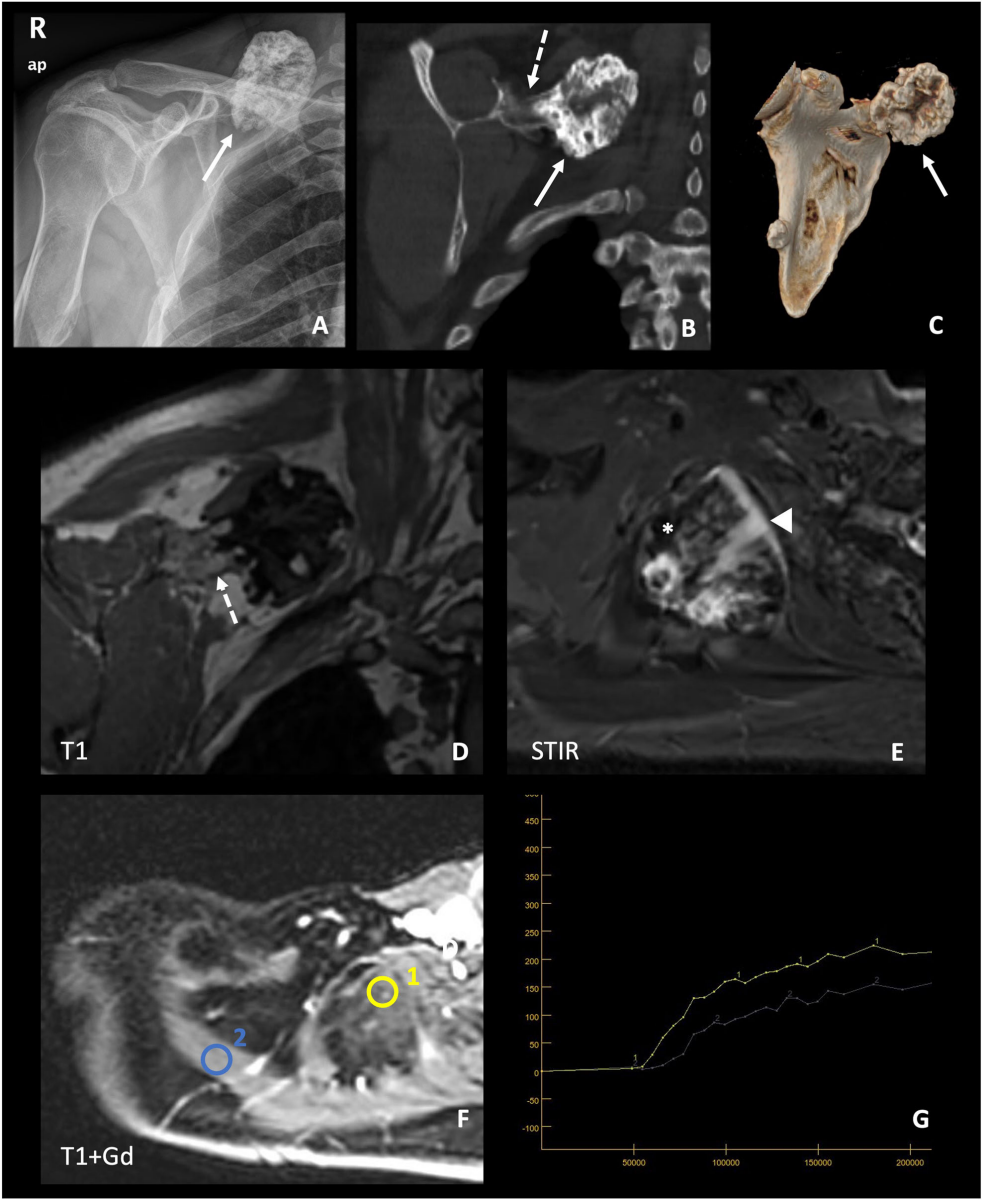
Detailed study of an osteochondroma in the right scapula of the 54-year-old male from Fig. 3. Targeted plain radiograph (A) and CT images: coronal reconstruction (B) and 3D volumetric reconstruction (C). MRI: T1W coronal plane (D), STIR axial plane (E), post-contrast dynamic study, with reference image (F), and curves of enhancement (G). Some characteristic features of the osteochondroma can be seen, such as the partially calcified cartilage cap with a ring-and-arc pattern (solid arrows). The cortical and medullary continuity with the underlying bone (dashed arrows) is key for making a precise diagnosis of osteochondroma. The cartilaginous cap shows high SI in STIR (arrowhead), with foci of calcification (low SI in STIR) (*). The cap thickness was 10 mm, not suspicious of malignancy, but surveillance was recommended. In the dynamic post-contrast study, the cartilaginous cap (ROI no. 1) presents an enhancement curve type II (progressive enhancement), which is similar to that of the ipsilateral supraspinatus muscle (ROI no. 2) and suggests a benign lesion
